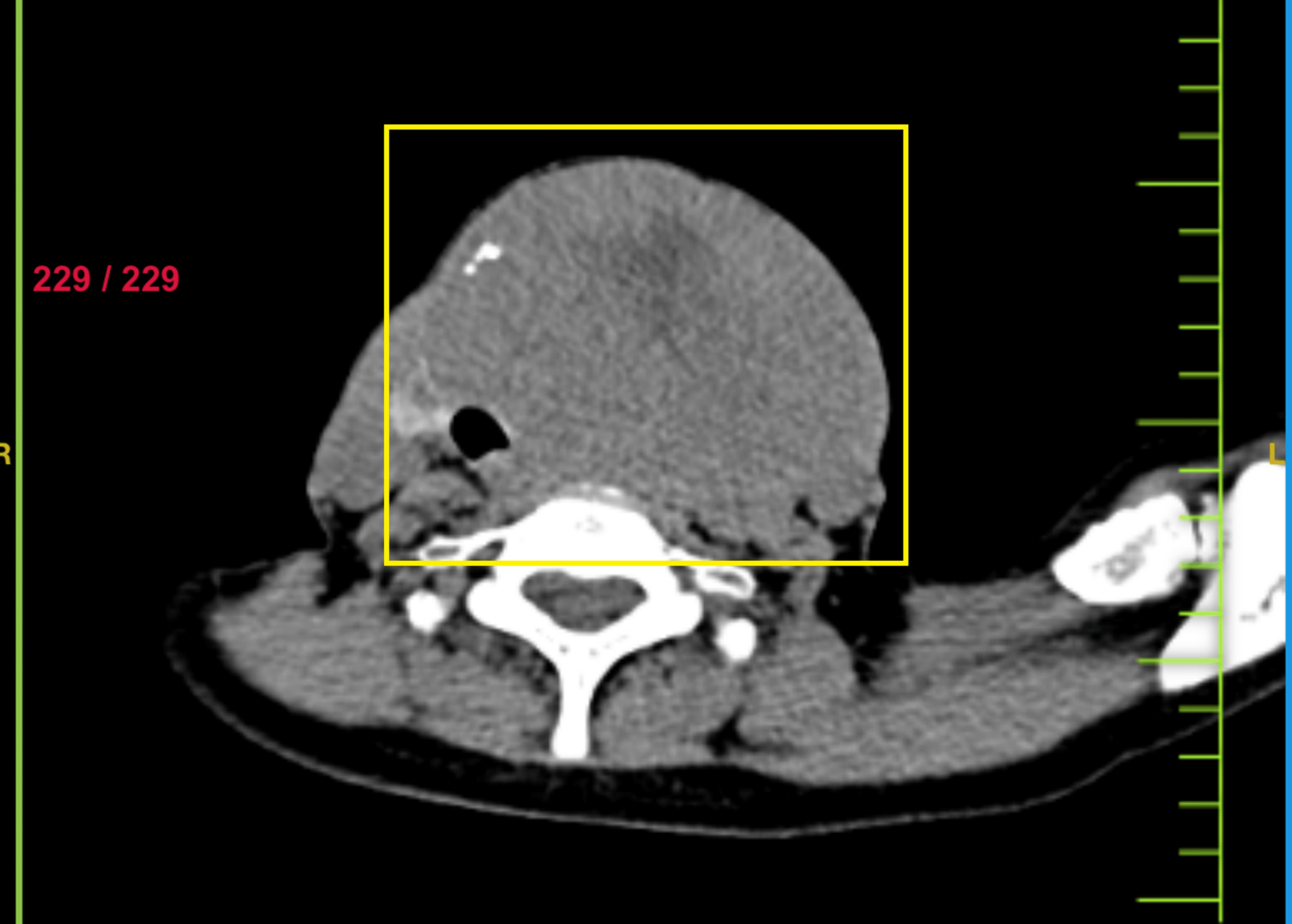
Non-contrast computed tomography image showing thyroid mass with tracheal deviation to the right
